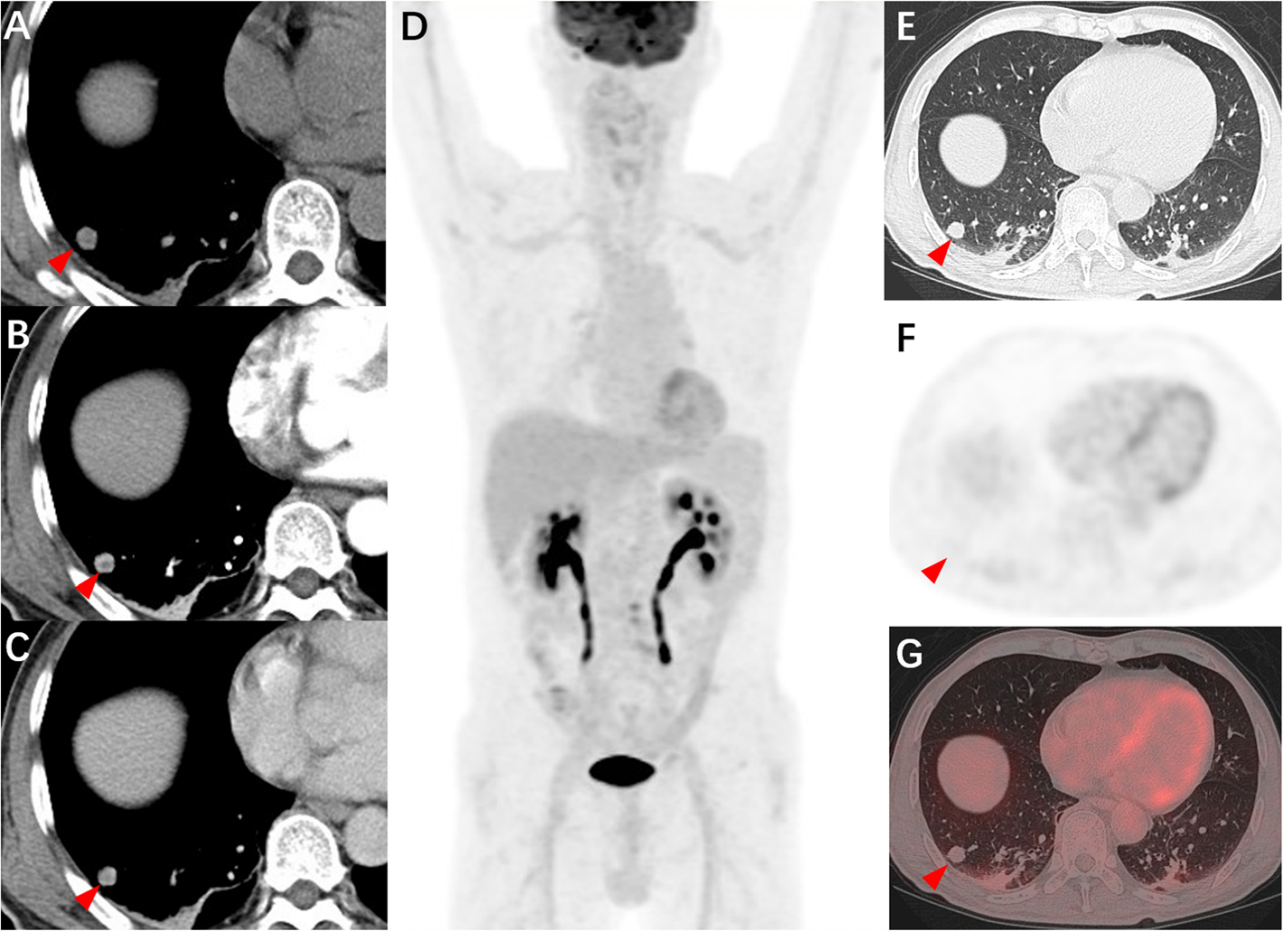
Transverse contrast-enhanced CT scan of the chest (A-C, red arrowheads) revealing a solitary solid peripheral pulmonary nodular in the right lower lobe with well-defined margins and mild centripetal enhancement. The maximum intensity projection PET Image (D) showing normal in the whole body. Transverse chest CT (E), corresponding PET (F) and fusion PET/CT (G) images demonstrating a solitary pulmonary nodular in the right lower lobe with no increased uptake of ^18^F-FDG
